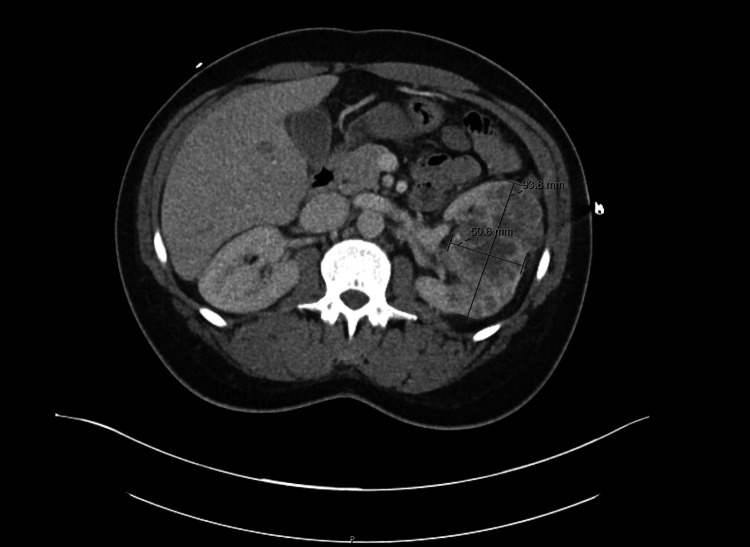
A CT scan of the abdomen shows a left renal mass
